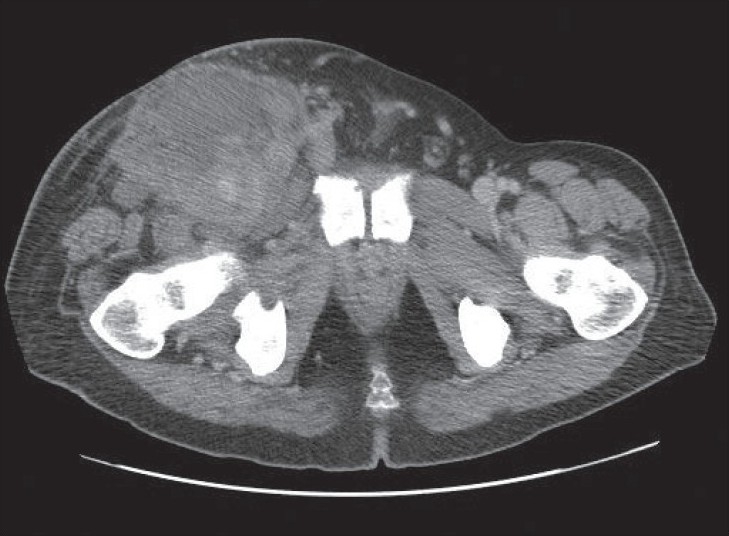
CT image showing mass extending into the right side of the pelvis
